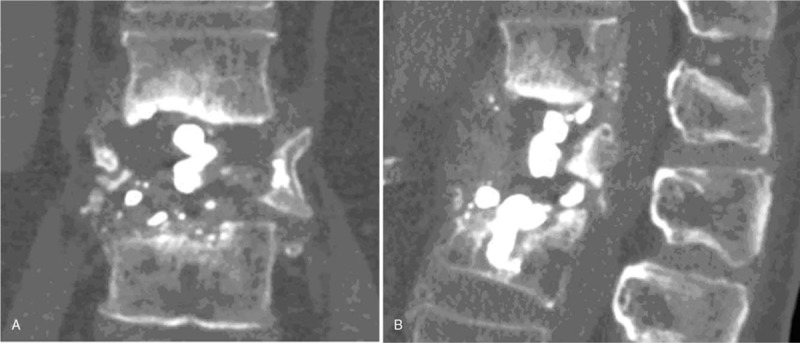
Computed tomography reconstruction of the L1 vertebra showing vertebral collapse of L1 after vertebroplasty, with broken PMMA pieces embedded in the L2 vertebra. PMMA = polymethylmethacrylate.
